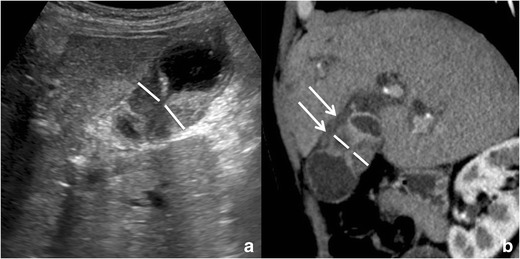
Gallbladder cancer: gallbladder adenocarcinoma may involve the gallbladder wall with various patterns. This case of gallbladder adenocarcinoma with annular involvement (*white lines*) can be differentiated from an adenomyomatosis because of the absence of cystic spaces (RAS) within the wall thickening on ultrasound (a) and because of the presence of hypodense tissue between the gallbladder wall and the adjacent liver (*arrows*) on contrast-enhanced CT (b)
